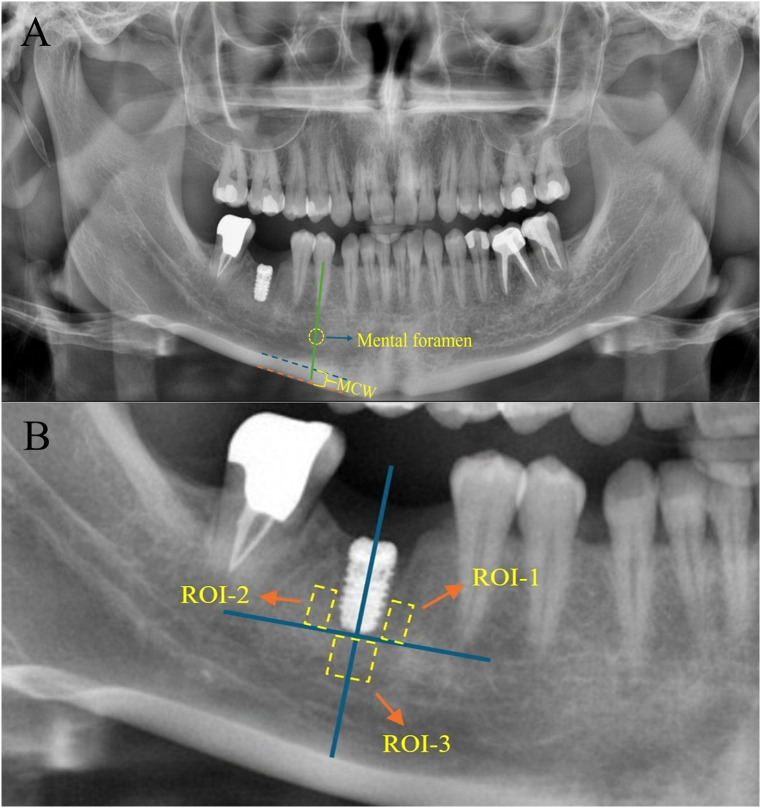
Standardized morphometric measurements and peri-implant ROI placement on panoramic radiography. (A) Morphometric measurements on panoramic radiography. The mental foramen was used as the anatomical reference point for mandibular cortical width (MCW) and mental length (ML) measurements. MCW was measured perpendicular to the inferior mandibular cortex, and PMI was calculated as the ratio of MCW to ML. (B) Enlarged view of peri-implant ROI placement. For fractal analysis, the blue longitudinal line indicates the implant long axis, and the blue transverse line indicates the perpendicular apical reference line passing through the implant apex. These geometric reference lines were defined on the 3-month radiograph and were used to reproduce the corresponding baseline ROI locations by side-by-side matching of stable anatomical landmarks. ROI-1 and ROI-2 were positioned in the closest available mesial and distal peri-implant trabecular bone adjacent to the implant surface, respectively, with their inferior borders aligned with the apical reference line. ROI-3 was positioned immediately apical to the implant apex, with its superior border aligned with the same apical reference line and centered on the implant long axis. The implant surface, implant threads, adjacent roots, cortical bone, mandibular canal, sinus floor, radiopaque restorations, and areas of anatomical superimposition or artifact were excluded from the ROIs.
